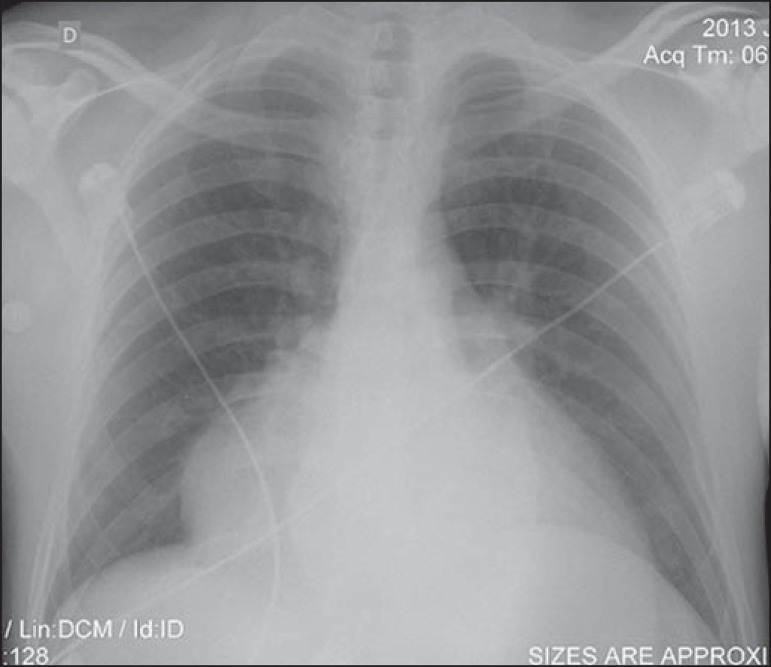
Chest X-ray showing a mass with well-defined borders. Pulmonary
